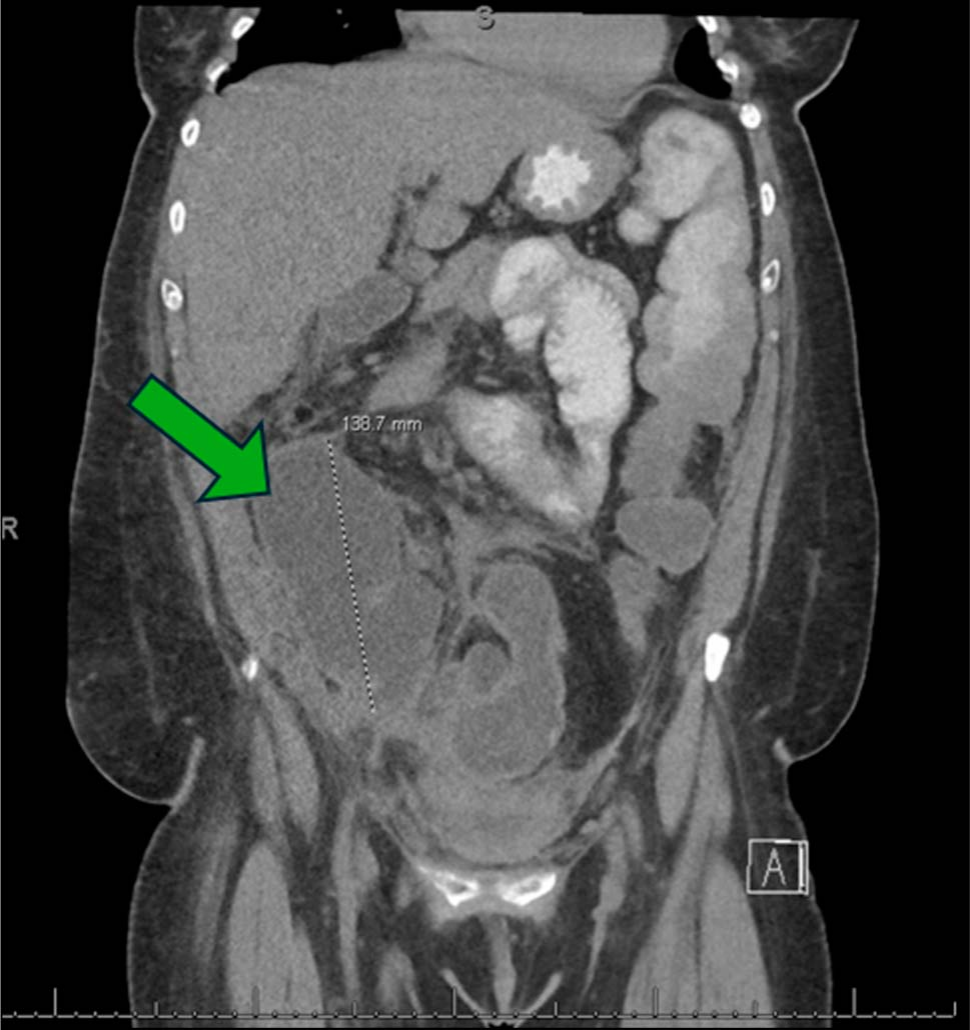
Computed tomography with oral and rectal contrast showing a complex, dilated fluid-filled tubular structure within the right lower quadrant, representing bowel, measuring 13.9 × 7.6 × 5.7 cm (green arrow).
